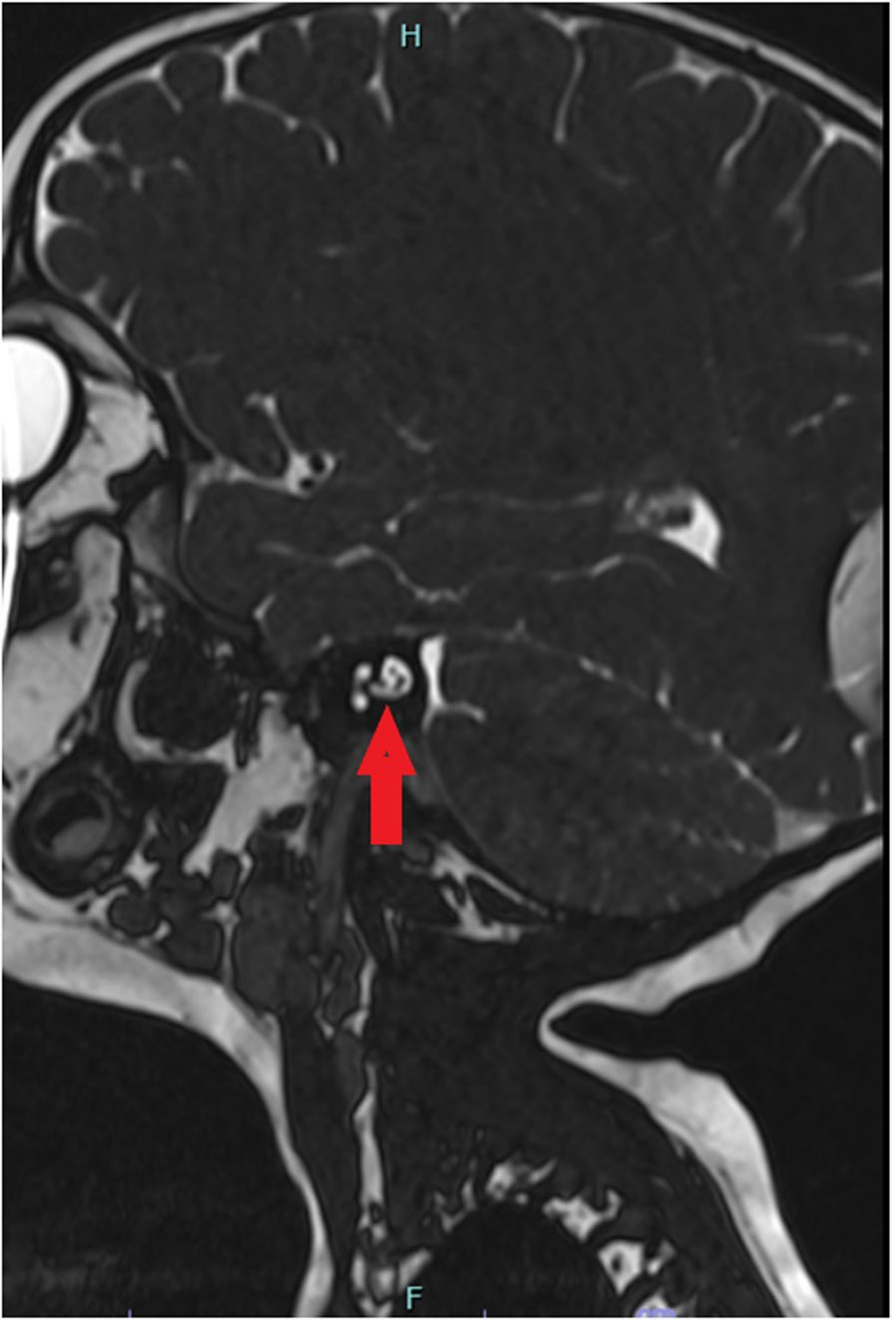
Sagittal T2 Magnetic Resonance Imaging of the head post- trauma. Sagittal T2 Magnetic Resonance Imaging of the head post-trauma with no signs of brain pathology, and the anatomy of the right 7^th^ and 8^th^ cranial nerves was normal (red arrow)
